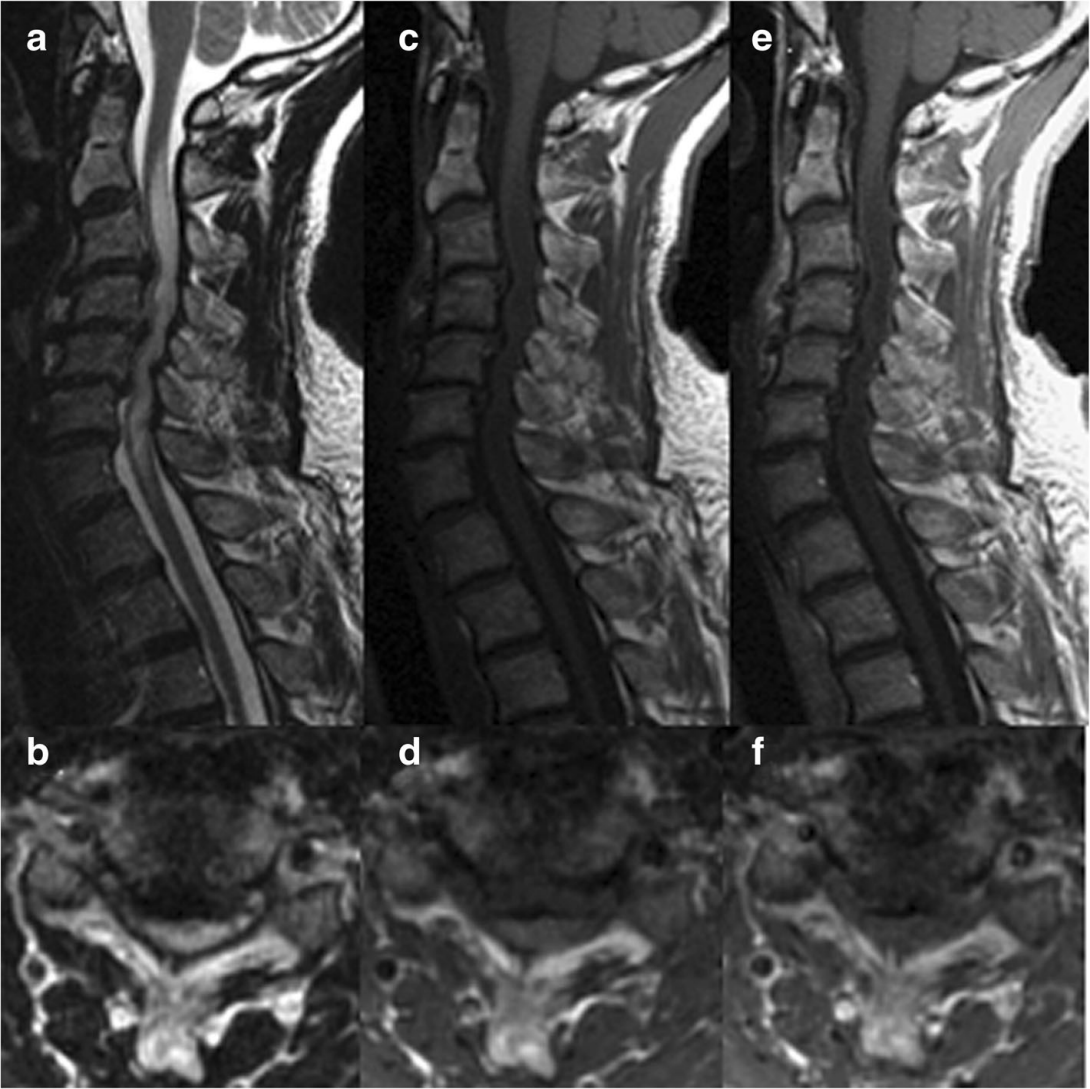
MRI images of a 67-year-old man with spinal cord edema due to cervical spondylosis revealing T2 high (a, b), T1 iso (c, d) signal intensity, and negative gadolinium enhancement (e, f)
